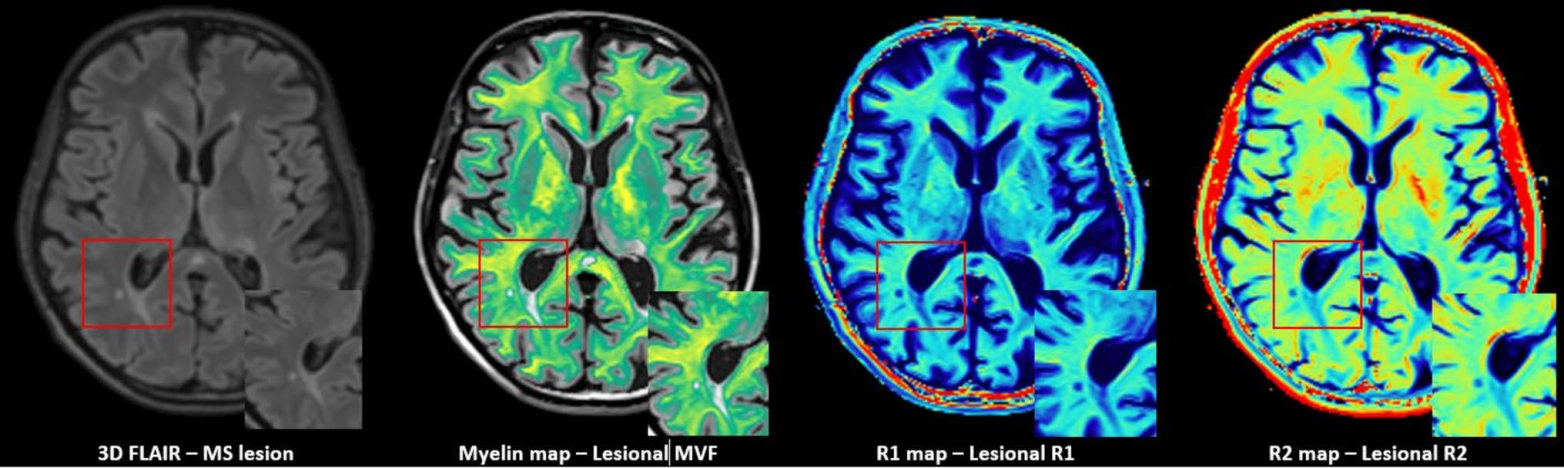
Conventional sagittal 3D FLAIR image and MDME-derived Myelin, R1 and R2 maps. Zoomed image of demyelinating lesions on the lower right corner
